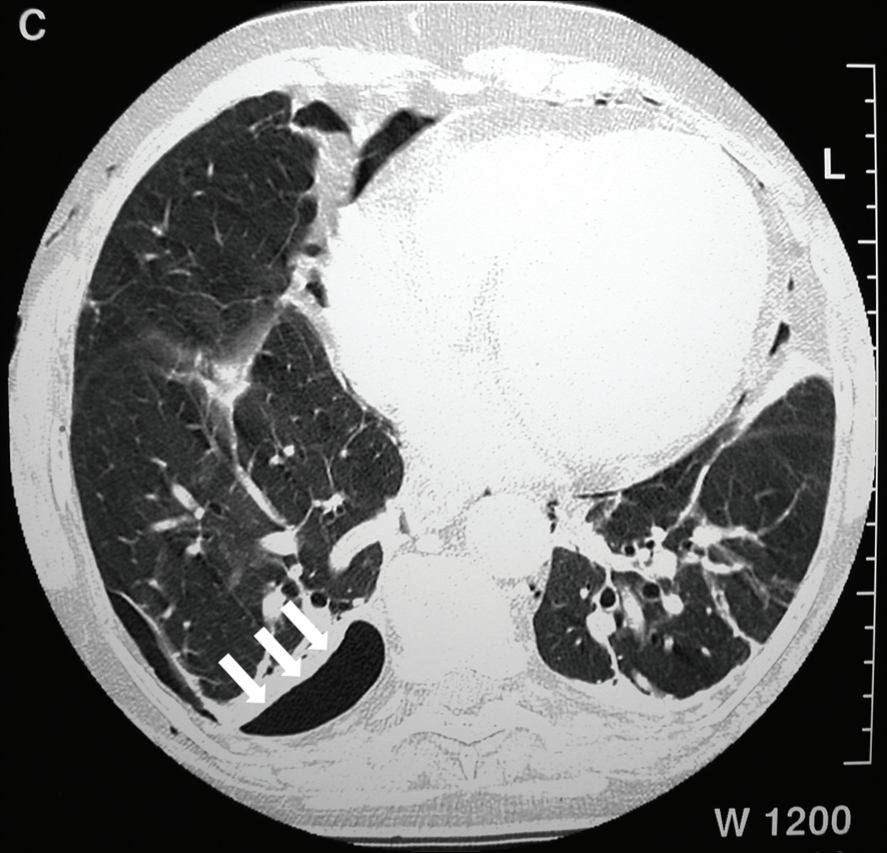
Thoracic computed tomography (CT) showing a small right-side pneumothorax (white arrows).
